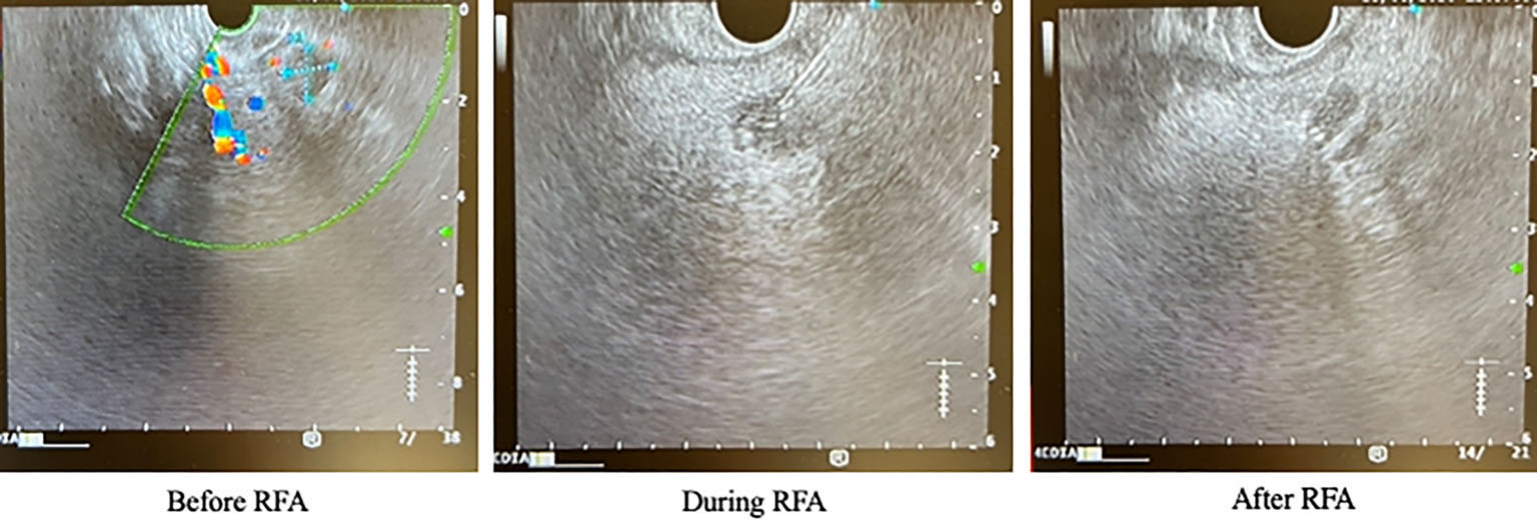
Endoscopic ultrasound images of the patient before, during and after RFA. RFA, radiofrequency ablation.
